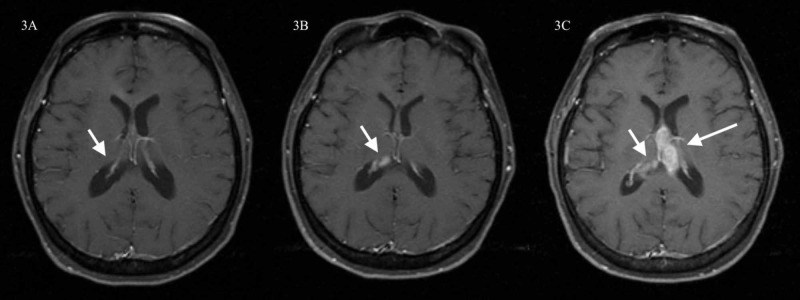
Post-contrast axial T1-weighted image MRI of the brain at two (Figure 3A, left), three (Figure 3B, center), and nearly five years (Figure 3C, right) from the time of diagnosis Post-contrast axial T1-weighted image MRI of the brain shows significant improvement of the lesion with almost disappearance of the periventricular enhancement two years after diagnosis, one year after completion of twelve rounds of temozolomide and six weeks of radiation. The hydrocephalus has resolved (Figure 3A, left). On brain MRI performed the following year, three years after diagnosis, a bilobed focus of enhancement is seen within the right ventricle. This represented the first clear imaging evidence of tumor recurrence (Figure 3B, center). Eighteen months later, brain MRI showed evident progression of the lesion (Figure 3C, right). At that time the patient declined further treatment. She died six months later, more than five years from the original diagnosis. MRI, magnetic resonance imaging.
